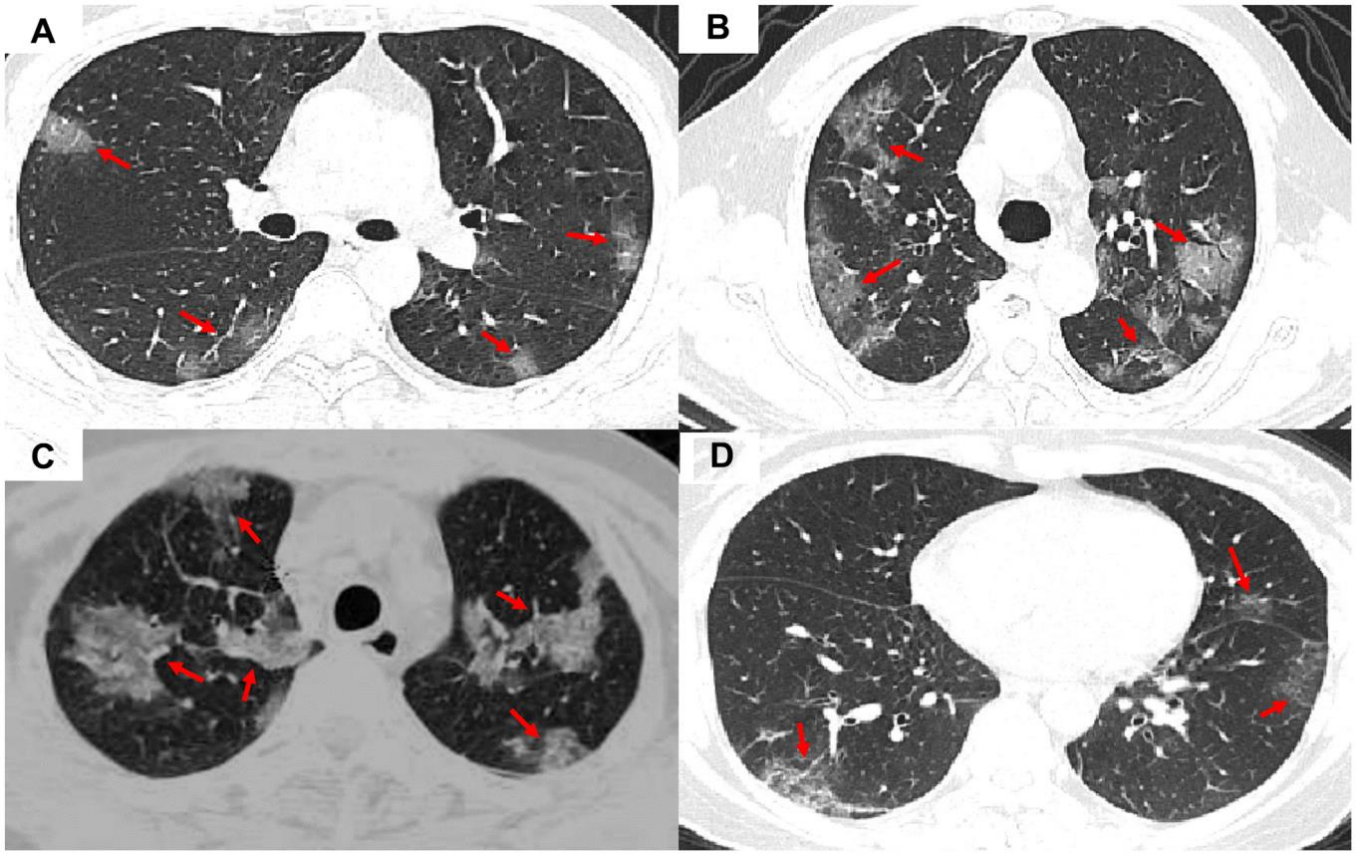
Representative images of COVID-19 pneumonia, adenovirus pneumonia, cytomegalovirus pneumonia, and influenza virus pneumonia. (A) A transverse CT image from a 35-year-old man with adenovirus pneumonia showing bilateral ground-glass opacities in the upper lobes with a rounded morphology (arrows). (B) COVID-19: A transverse CT image from a 57-year-old man with COVID-19 showing more limited ground-glass opacities in the bilateral upper lobes with an elliptical morphology (arrows). (C) A transverse CT image obtained in a 45-year-old female with cytomegalovirus pneumonia showing bilateral ground-glass and burr-like, denser, and less transparent distribution (arrows). (D) A transverse CT image of a 61-year-old man diagnosed with influenza virus pneumonia showing bilateral ground-glass opacities in the upper lobes (arrows).
